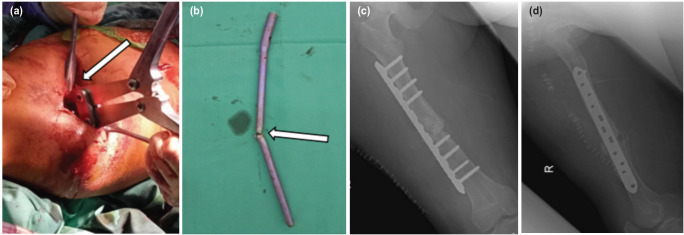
(a) Jumbo cutter was introduced at visible nail (b) and sectioned the nail in two parts with multiple bites. Appearance of complete extracted nail - two parts. (c, d) Immediate postoperative radiograph showing locking compression plate and bone graft was done after removal of bent nail.
